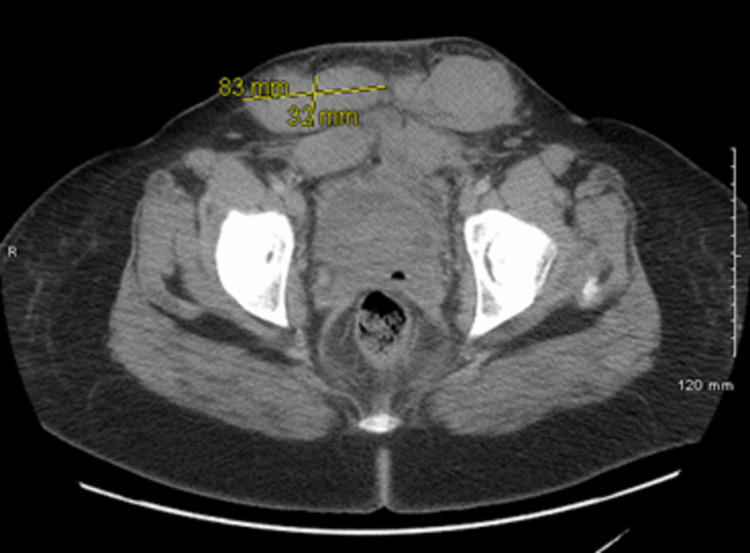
Computed tomography axial view showing hematoma of 8.3 cm x 3.2 cm in subcutaneous soft tissue
